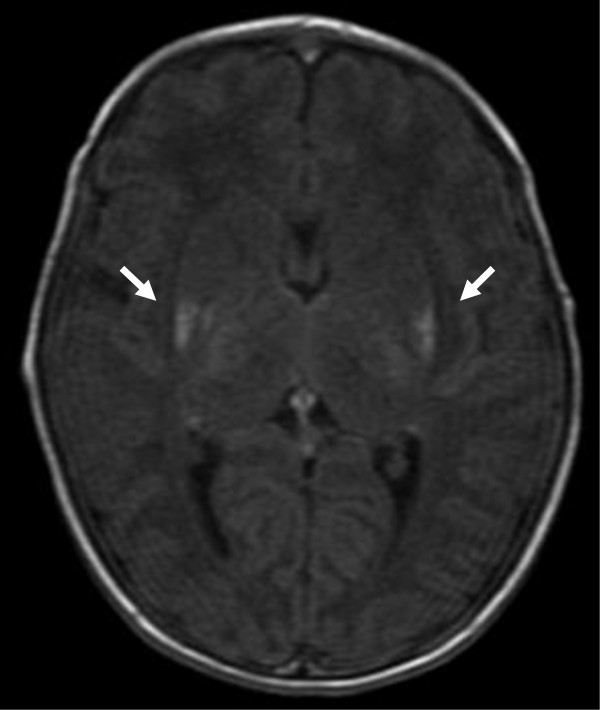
Brain head magnetic resonance reveals bilateral small hyperintensities on T1-weighted images in the basal ganglia: bilateral putamen, pallidum and thalamus (white arrow).
